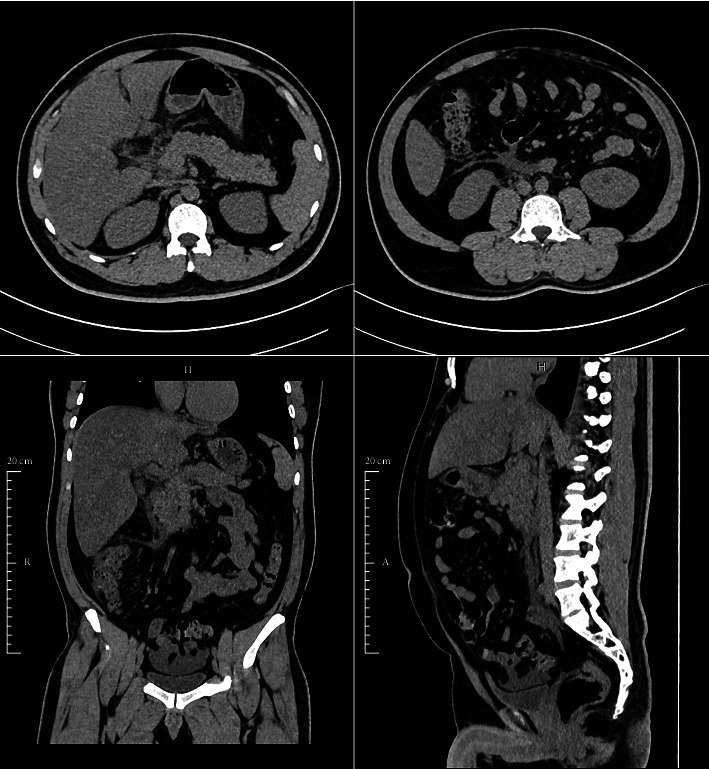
Noncontrast abdominal CT showing an enlarged pancreas, peripancreatic fat stranding, and peripancreatic fluid collection, consistent with Balthazar score D.
